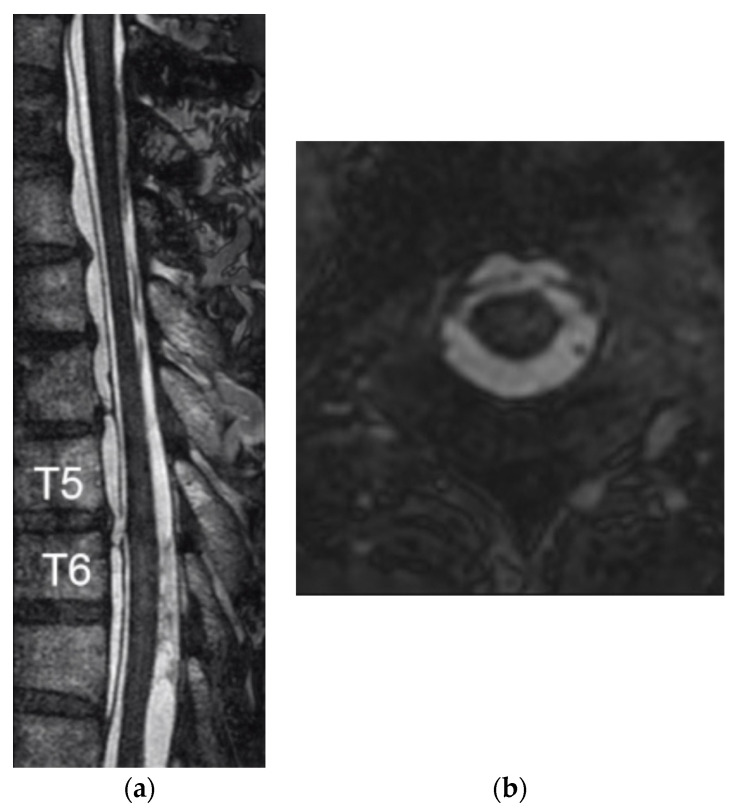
A balanced turbo field echo (BTFE) MRI sequence, which achieves rapid and high-resolution imaging of the spinal anatomical structures, reveals a gap in the anterior dura mater at the T5–T6 level. (a) sagittal-, and (b) axial-oriented images.
